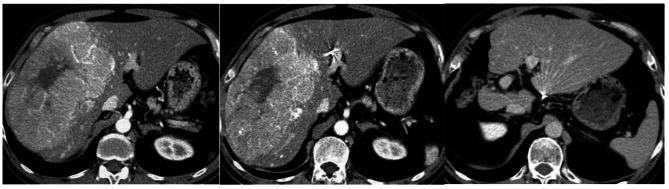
A 54 year-old male advanced HCC patient. A: CT image showed a huge HCC (16 cm) in the right lobe liver with right segmental portal vein invasion (not shown). B: After 2 courses of HAIC neoadjuvant therapy with SD, this patient underwent right hepatectomy. C: 10-year follow-up CT image showed no evidence of intrahepatic recurrence
